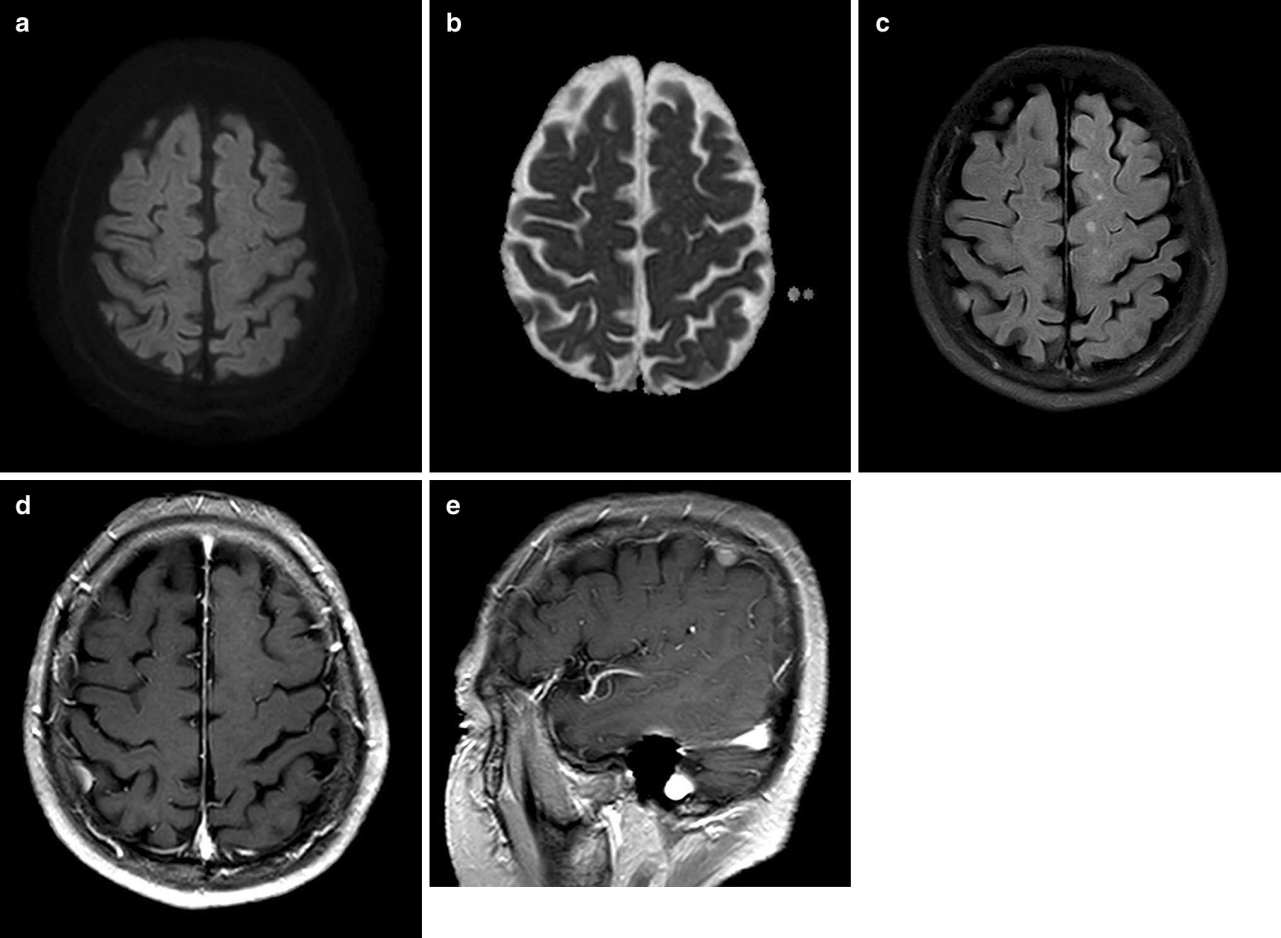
False-negative case. A 68-year-male patient with sudden onset vertigo. a, b Small extraaxial mass is located in the right high parietal convexity without diffusion restriction. c The lesion exhibits isointensity on FLAIR image. However, all residents interpret DWI as normal. d, e On contrast-enhanced axial (d) and sagittal (e) T1-weighted images from the following day, the lesion exhibits homogenous enhancement, suggesting convexity meningioma
